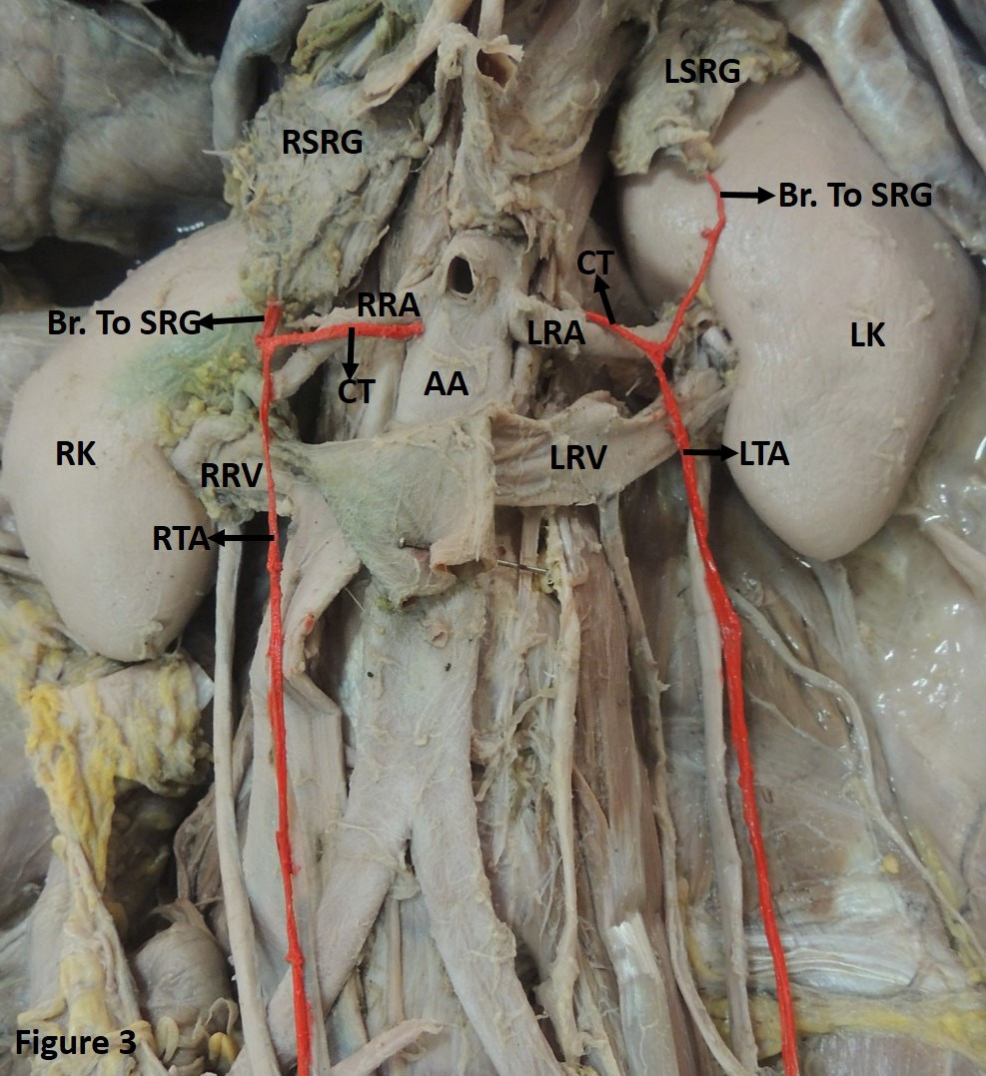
Showing a common arterial trunk (CT) arising from the renal artery (LRA) and abdominal aorta (AA) on the left and right sides respectively. The CT then bifurcates to provide a suprarenal branch (Br. To SRG) and further continues as the testicular artery proper (TA). Both the TAs (LTA and RTA) are seen to cross the respective left and right renal arteries (LRA and RRA) and renal veins (LRV and RRV) in this process. LSRG and RSRG: left and right suprarenal gland; LK and RK: left and right kidney.
